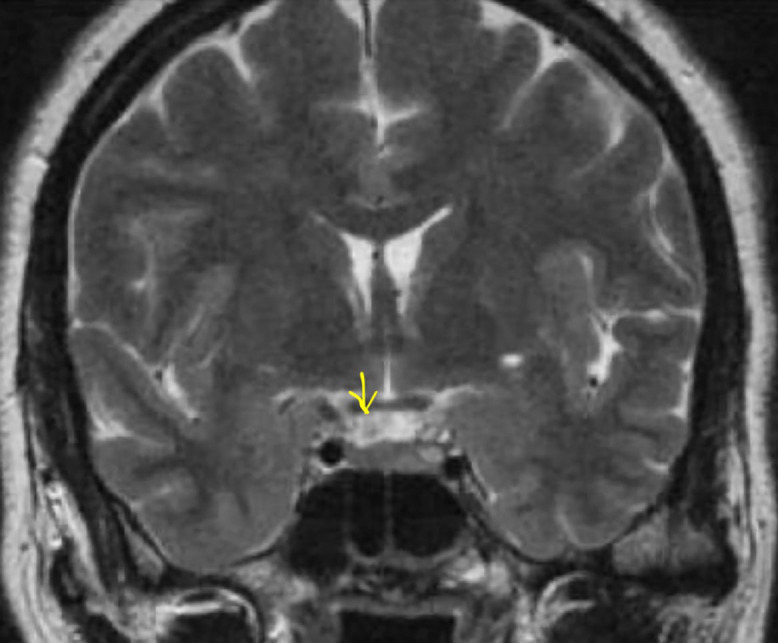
T2 weighted magnetic resonance imaging of brain with pituitary cuts showing isointense lesion (yellow arrow) in the pituitary gland suggestive of pituitary microadenoma (Magnification:300 DPI).
